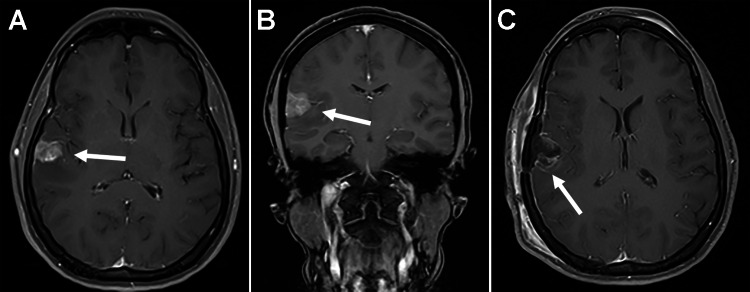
Magnetic resonance imaging (MRI) of the brain. (A) Axial and (B) coronal T1-weighted postcontrast MRI scans demonstrating a 1.3×1.8×1.7 cm right temporal mass. (C) Postoperative axial postcontrast MRI demonstrating gross total resection with no acute postsurgical complications and scant blood products in the resection cavity.
